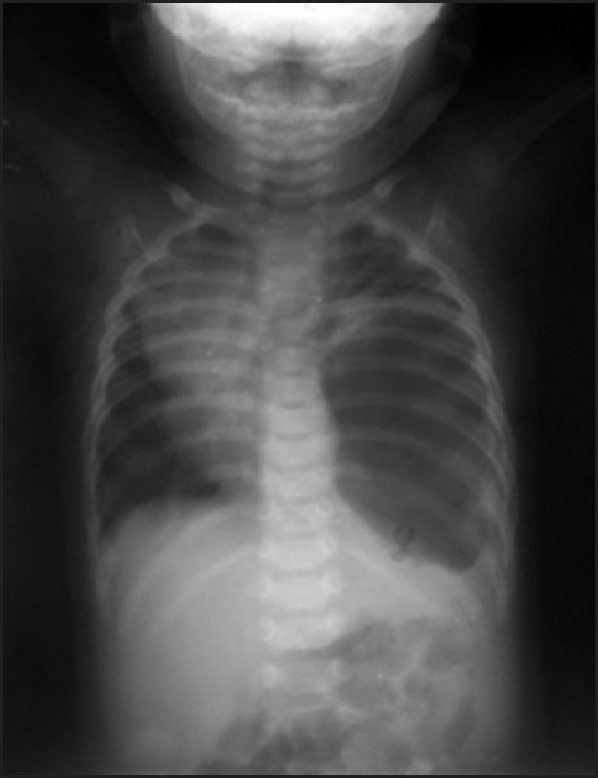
Plain chest X-ray masquerading as a tension pneumothorax on the left side with mediastinal shift on the right side
